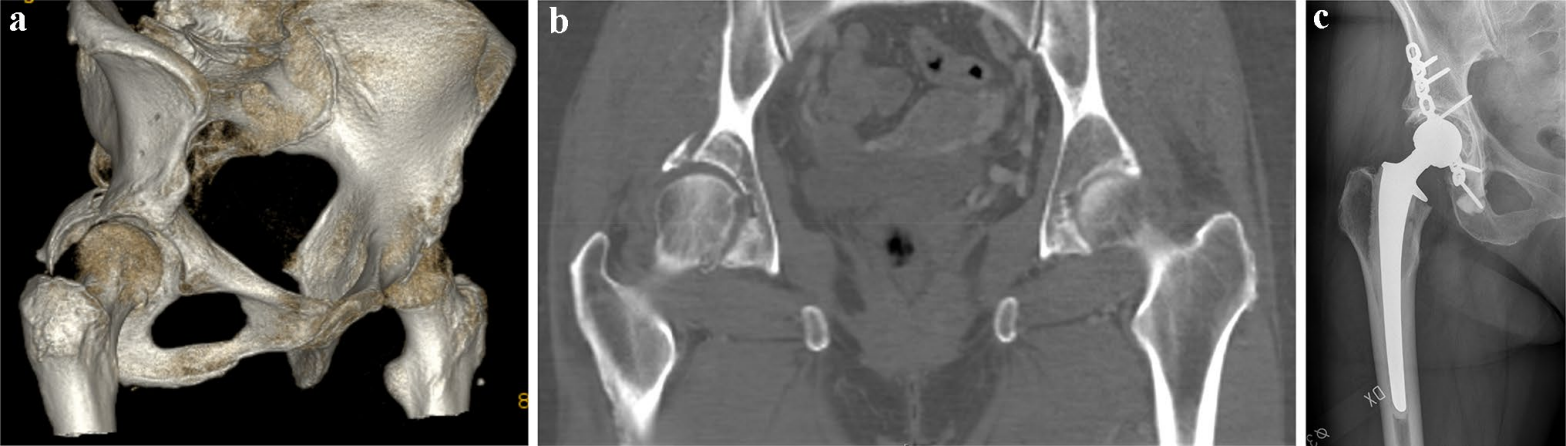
a 3D-CT scan in a 73 years old female after a traffic accident, displaying a Type 4 Pipkin fracture. b CT scan in the same patient displaying the fracture of the femoral head. c Postoperative x-ray in the same patient after plate fixation of the acetabular fracture and a cemented total hip arthroplasty
